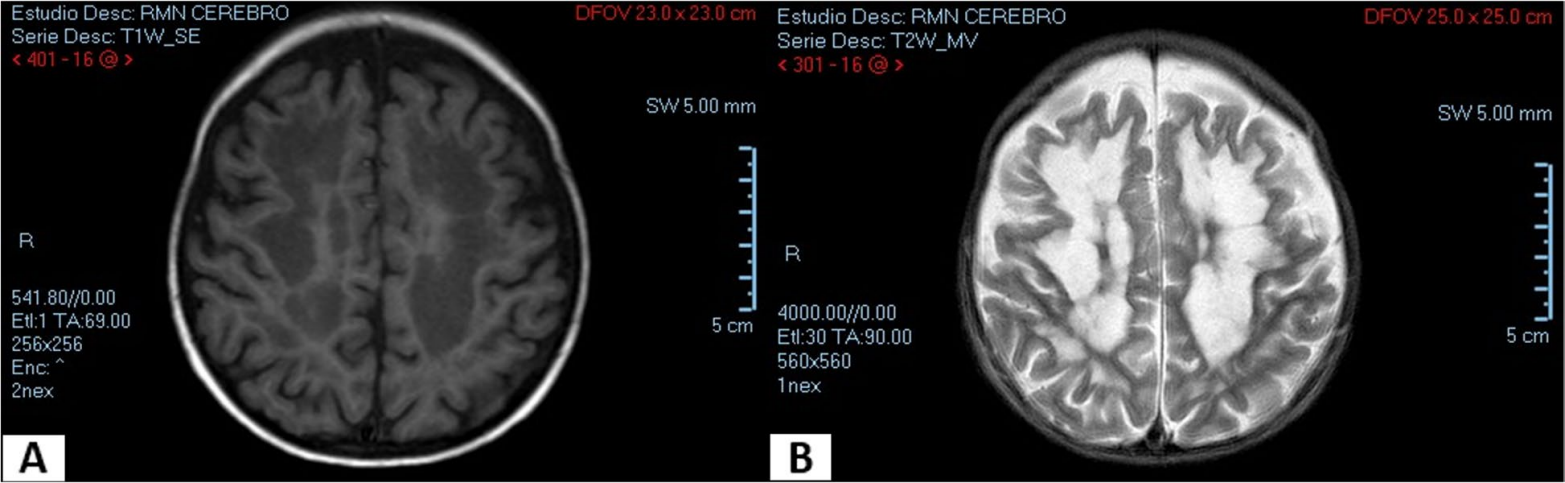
Magnetic resonance imaging. Cross-sectional area of the brain of the patient with Costello syndrome. Infra and supratentorial white matter involvement. A T1-weighted sequence. B T2-weighted sequence
